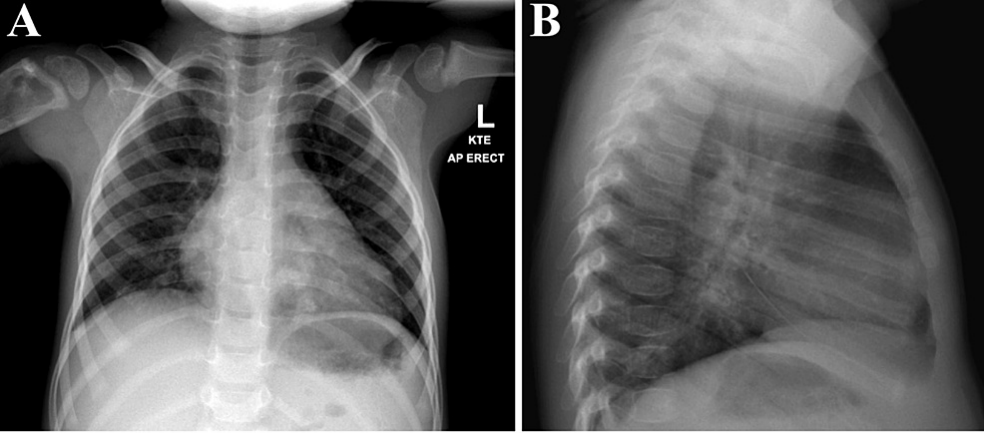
X-ray of the chest in the AP (A) and lateral (B) views AP: Anteroposterior
